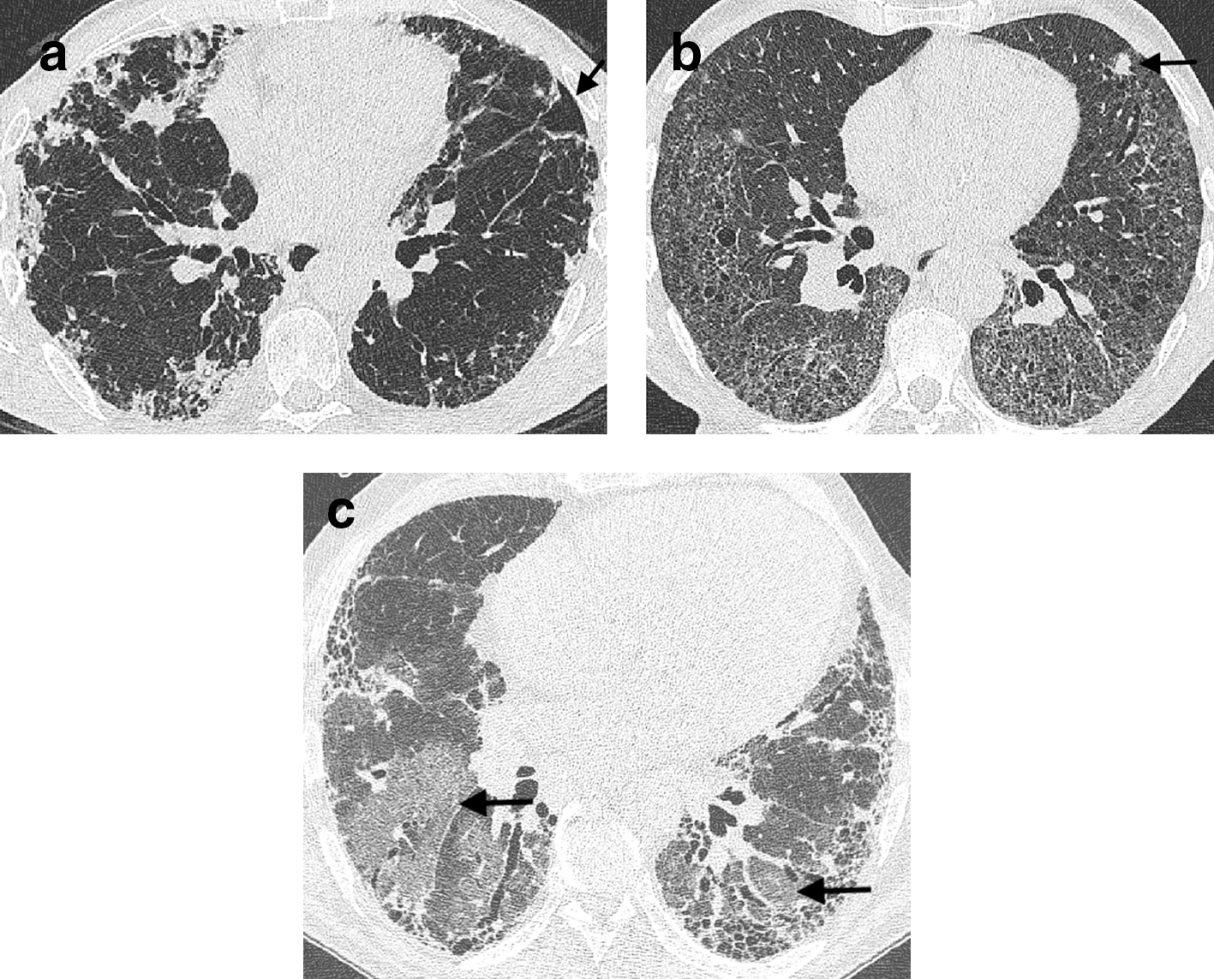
Important complications to identify on a CT in a patient with a fibrosing lung disease. A pneumothorax (a) can present as acute shortness of breath (arrow). Newly developing lung nodules (arrow) may represent early lung cancer which has a higher likelihood of developing, in patients with fibrosing lung disease (b). Widespread ground glass density occurring in normal regions of lung (arrows) should raise the possibility of an acute exacerbation of disease (c).
